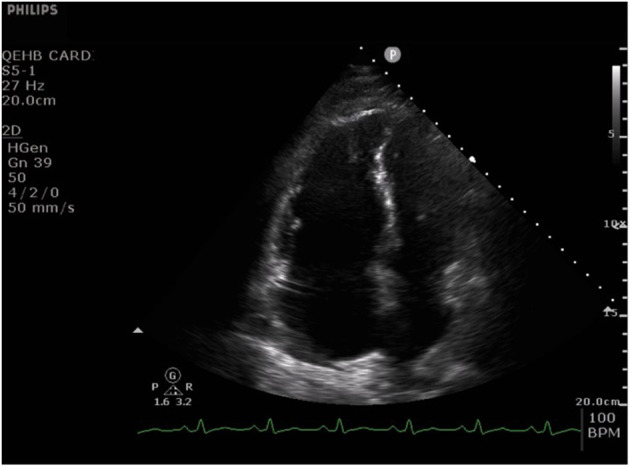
Apical four chamber echocardiographic still image from a cine typical of many patients with acute severe COVID-19 pneumonia. The cine (Video 1) shows a dilated right ventricle and severe impairment of radial right ventricle systolic function with relative preservation of long axis function. The left ventricle is small and hyperdynamic. There is also paradoxical septal wall motion and a thin rim of pericardial effusion adjacent to the right atrium.
